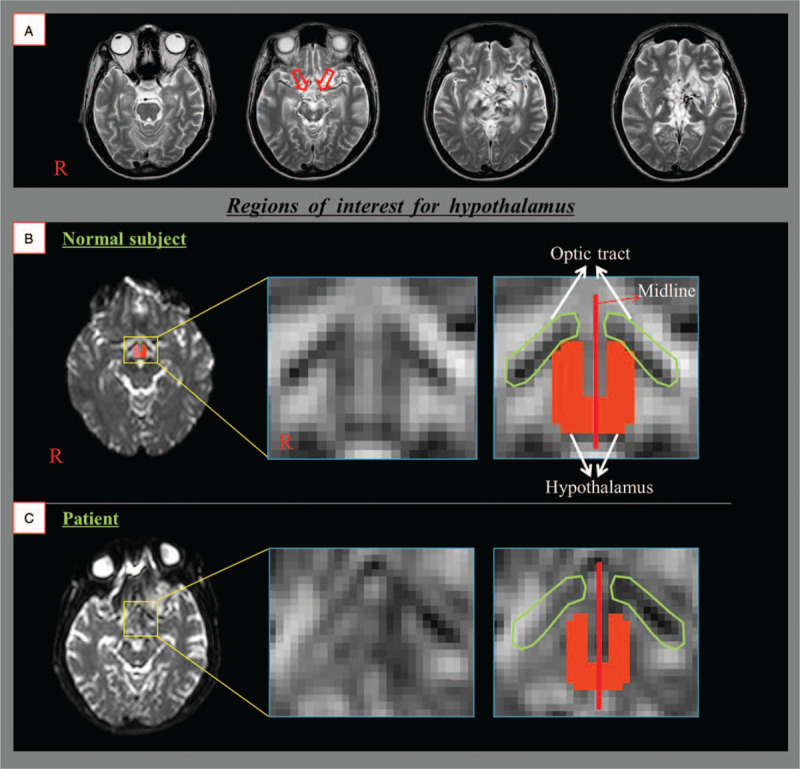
(A) T2-weighted brain magnetic resonance images show leukomalactic lesions in the hypothalamus (arrows), bilateral medial temporal lobe, and bilateral basal ganglia. (B) Regions of interest for the hypothalamus were localized by using the optic tract (anterior boundary), the mammillary body (posterior boundary), and the midline (medial boundary) at the level of the upper midbrain in the patient (C) and a representative normal subject.
